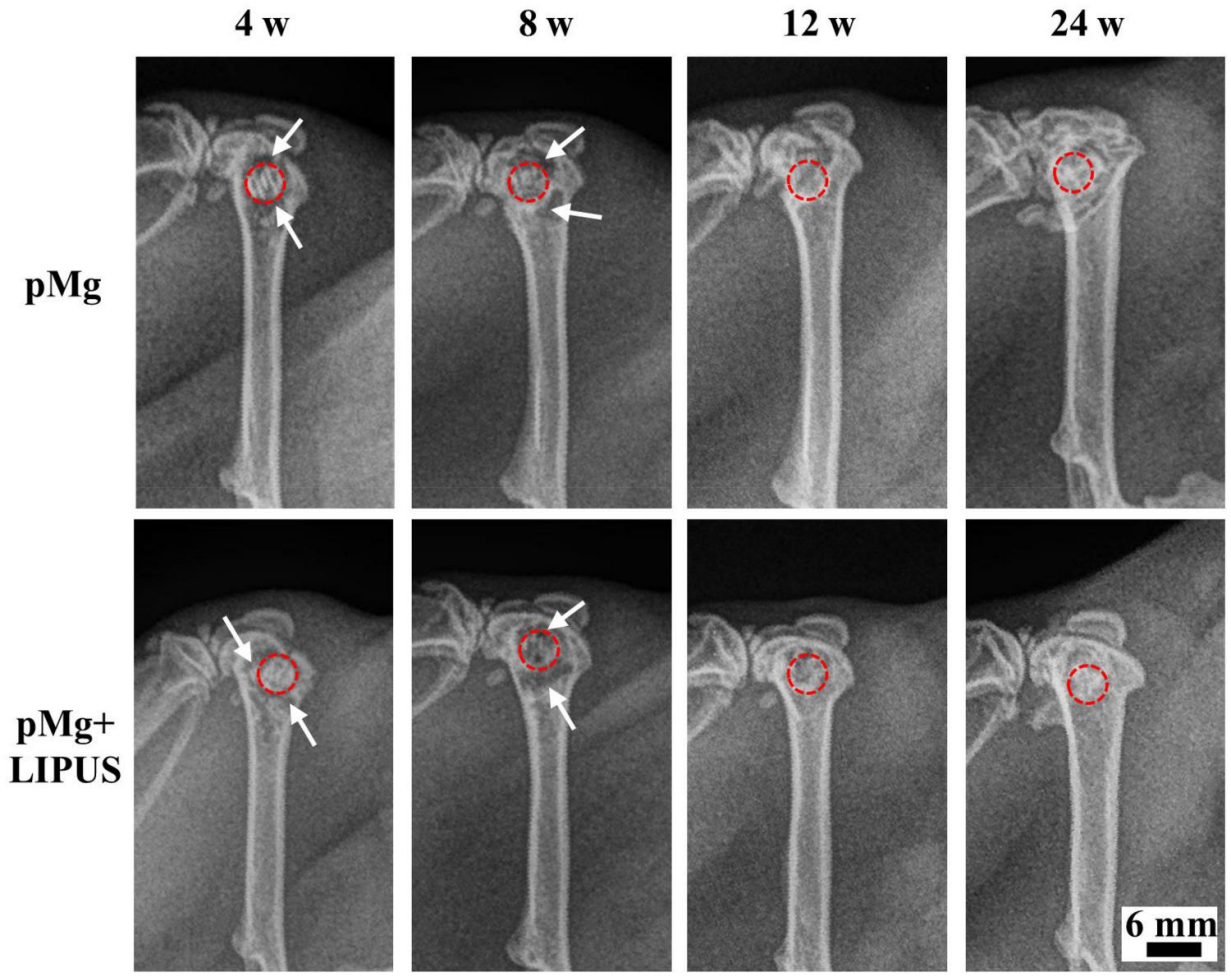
Representative radiographic images at 4, 8, 12 and 24 weeks after implantation. Arrows: low-density shadows, Rings: scaffold outline.
